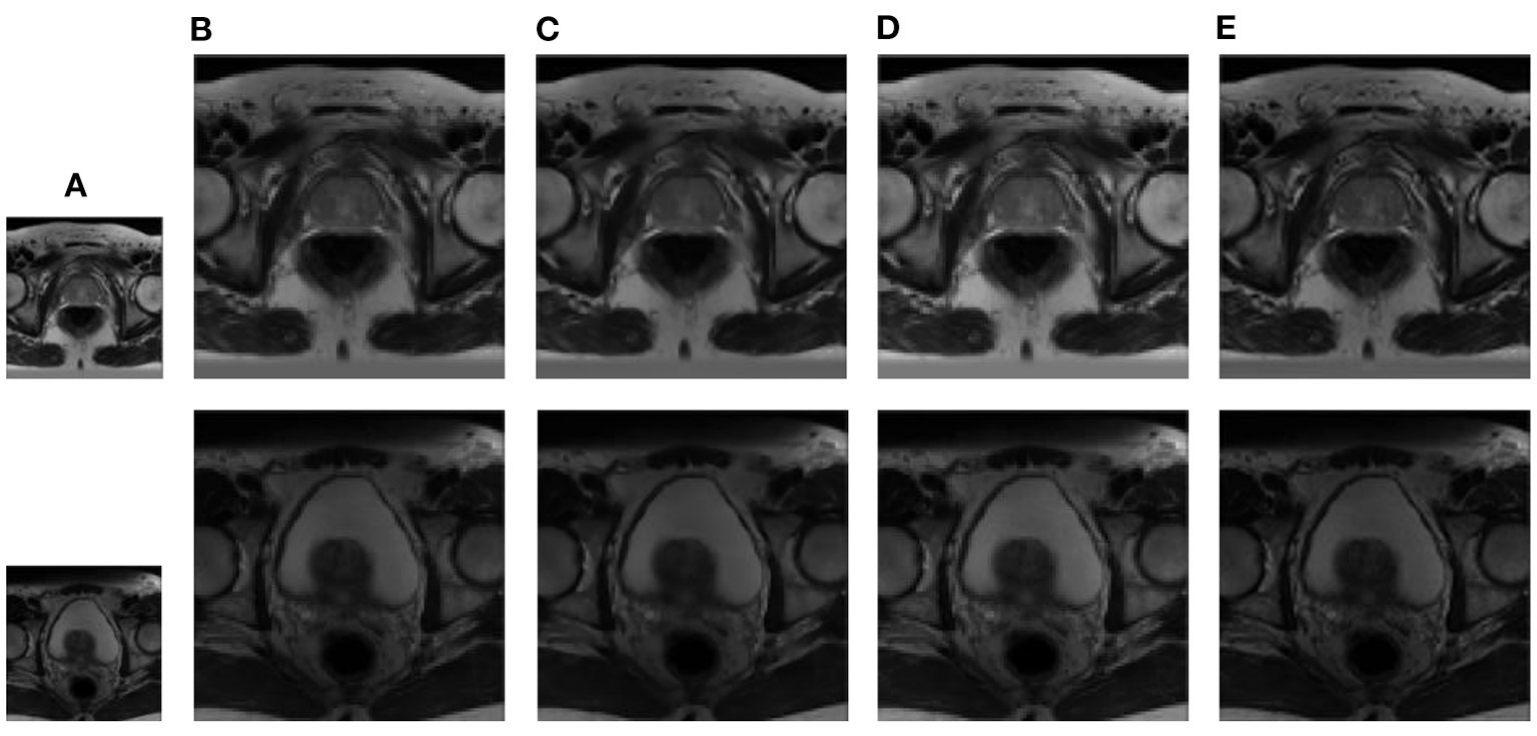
Reconstruction results of prostate MRI data from NCI-ISBI 2013 database: (A) LR band with downsampling factor 2, (B) NN, (C) LRTV, (D) TF-SISR, (E) NNTDTV.
